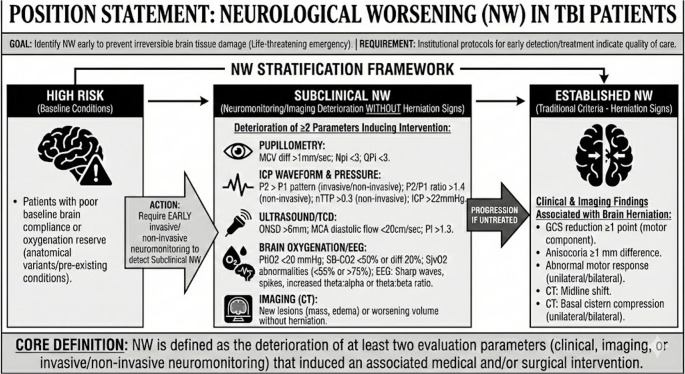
Proposed stratification model to expand the NW definition. NW: neuroworsening; GCS: Glasgow coma scale; CT: computed tomography; NPI: Neurologic pupillary index; QPi: Quantitative Pupillary Index, ICP: intracranial pressure; PbtiO2: parenchymal brain oxygen pressure; SvJO2: venous jugular oxygen saturation; NIRS: near infrared oxygen saturation; EEG: electroencephalographye*Established NW is defined by traditional criteria (clinical and imaging findings associated with brain herniation)*,* including*:*Glasgow Coma Score (GCS) reduction of at least 1 point in the motor component.Anisocoria of at least 1 mm of difference.Abnormal motor response (unilaterally or bilaterally).Midline shift on computed tomography (CT) imaging.Basal cistern compression (unilaterally or bilaterally) on CT imaging.*f*Subclinical NW is defined as the deterioration of at least two parameters in neuromonitoring (invasive or non-invasive)*,* or imaging (without herniation signs)*,* inducing medical and/or surgical interventions. These parameters include*:*Pupillometry maximum contraction velocity (MCV) difference >1 mm/sec between eyes.Neurologic pupillary index (Npi) <3.Quantitative pupillary index (QPi) <3.Optic nerve sheath diameter (ONSD) >6mm on any side evaluated by ultrasound.Peak 2 (P2) >Peak 1 (P1) pattern on any side of the skull during invasive or non invasive intracranial pressure (ICP) waveform analysis.P2/P1 ratio analysis on non-invasive ICP monitoring > 1.4 on any side of the skull.Normalized time to peak (nTTP) > 0.3 on any side of the skull during non invasive ICP monitoring.ICP >22mmHg.Medial cerebral artery (MCA) diastolic flow velocity < 20cm/sec on any side during transcranial Doppler (TCD) monitoring.Pulsatility index (PI) > 1.3 on any side during TCD monitoring.Sharp waves, spikes, or spike-and-wave complexes or increased theta: alpha ratio or theta: beta ratio in any area during EEG monitoring.Brain oxygen tissue pressure (PtiO2) < 20 mmHg on any side of the skull.Near infrared spectroscopy-based cerebral oxygen saturation (SB-CO2) < 50% at any side of the skull or a difference of 20% of SB-CO2 between sides.Jugular oxygen saturation (SjvO2) or near infrared spectroscopy-based jugular saturation (SB-SjvO2) <55% or >75% at any side of the head/neck.When neuromonitoring is not available, follow-up Imaging (CT scan) showing the presence of new lesions (mass, edema) or worsening of previous ones (increased volume of lesion or edema).*g*High-risk phenotypes for NW include patients with baseline conditions associated with poor brain compliance/brain oxygenation reserve (anatomical variants or pre-existing conditions) specified in* Table 1. *These patients will require early invasive/non-invasive neuromonitoring to detect subclinical NW after TBI*,* and do not delay action for managing it before additional tissue damage occurs.*
